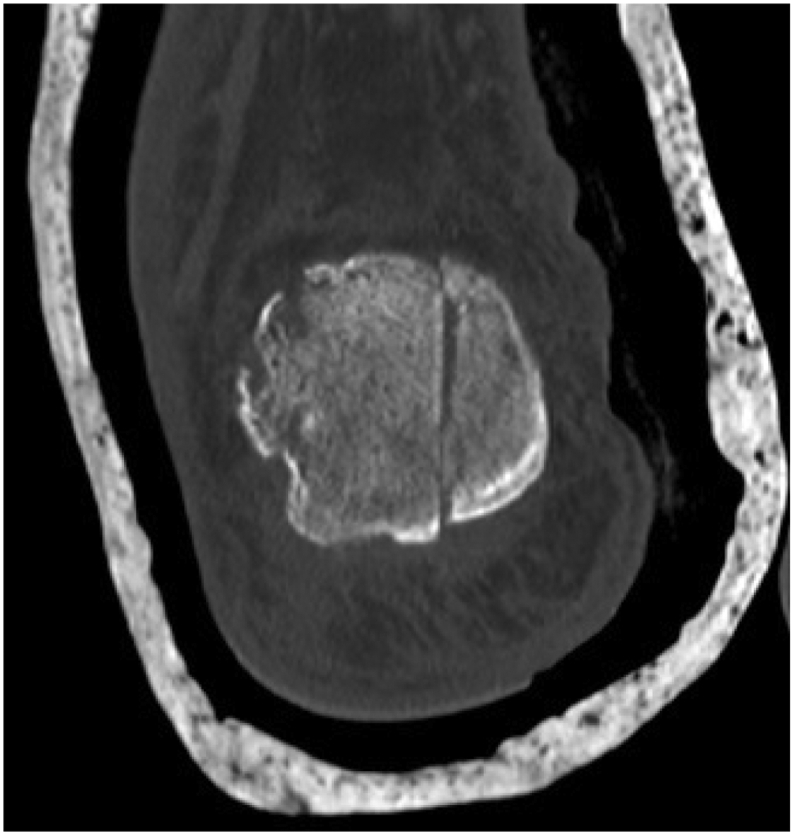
Post-operative coronal CT-slide showing the closing wedge calcaneal osteotomy.
